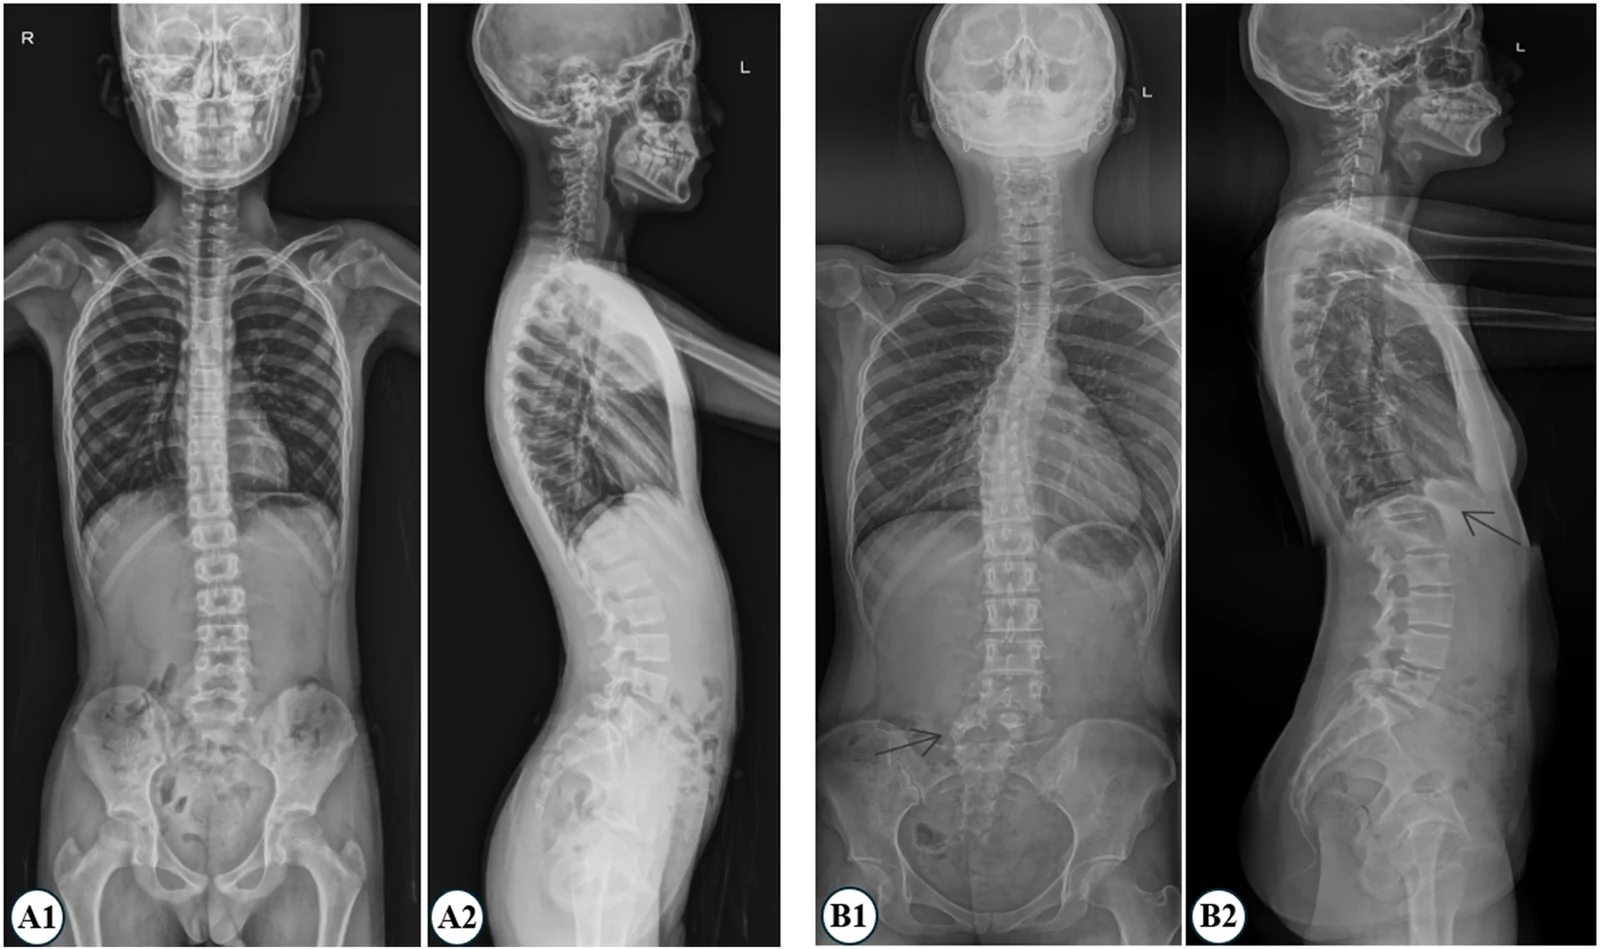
Representative full-spine radiographs obtained with a large-area flat-panel detector (FPD) and a conventional FPD. (A1, A2), anteroposterior and lateral radiographs acquired with the large-area FPD in a single exposure (image quality score, 4 for both). (B1, B2), anteroposterior and lateral radiographs acquired with the conventional FPD by segmented acquisition and image stitching (image quality score, 1 for both). Arrows indicate stitching misregistration artifacts.
